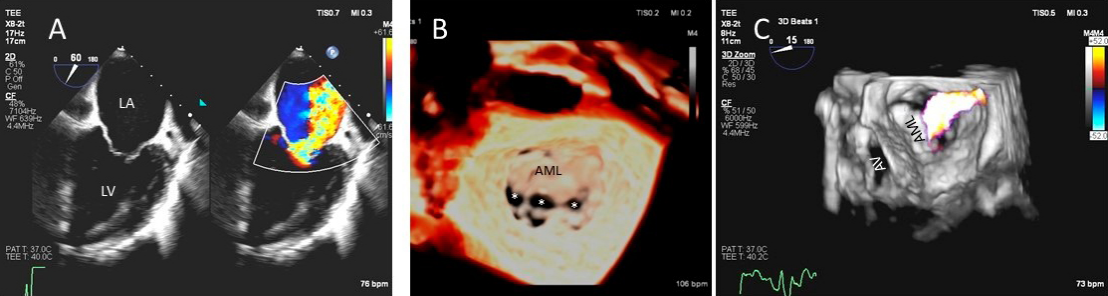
Functional Mitral regurgitation. (A) Transesophageal
